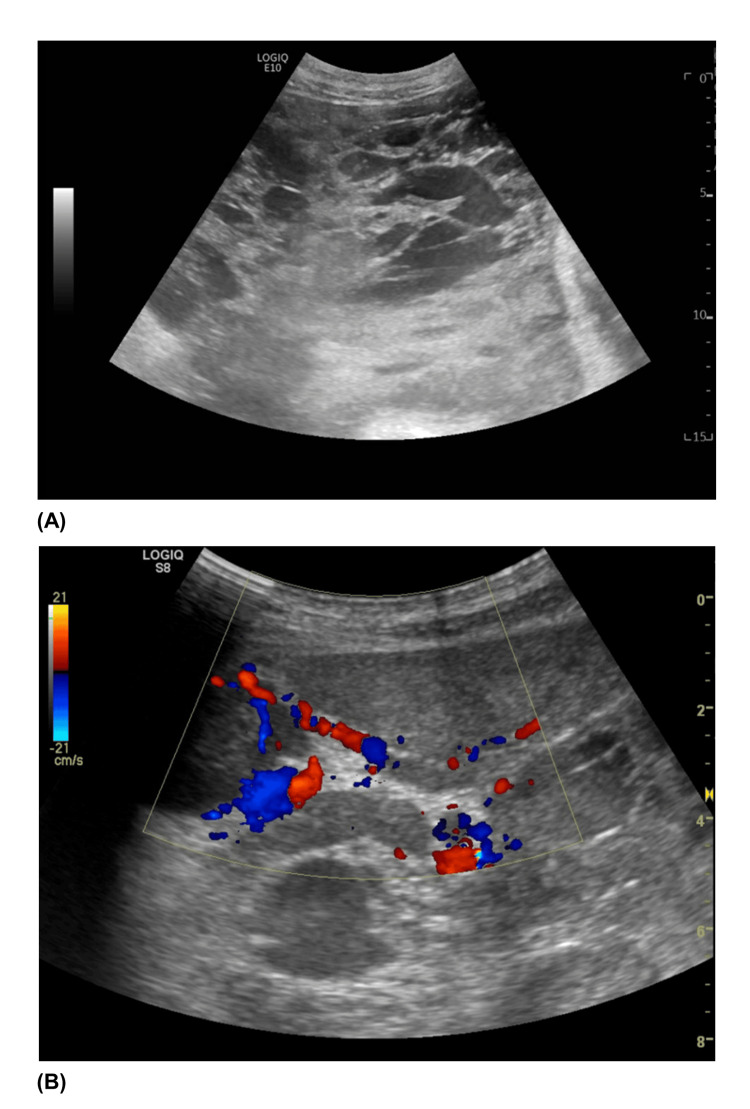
Abdominal ultrasonography. (A) B-mode; (B) Doppler, showing very vascularized nodularity in the peritoneal cavity.
